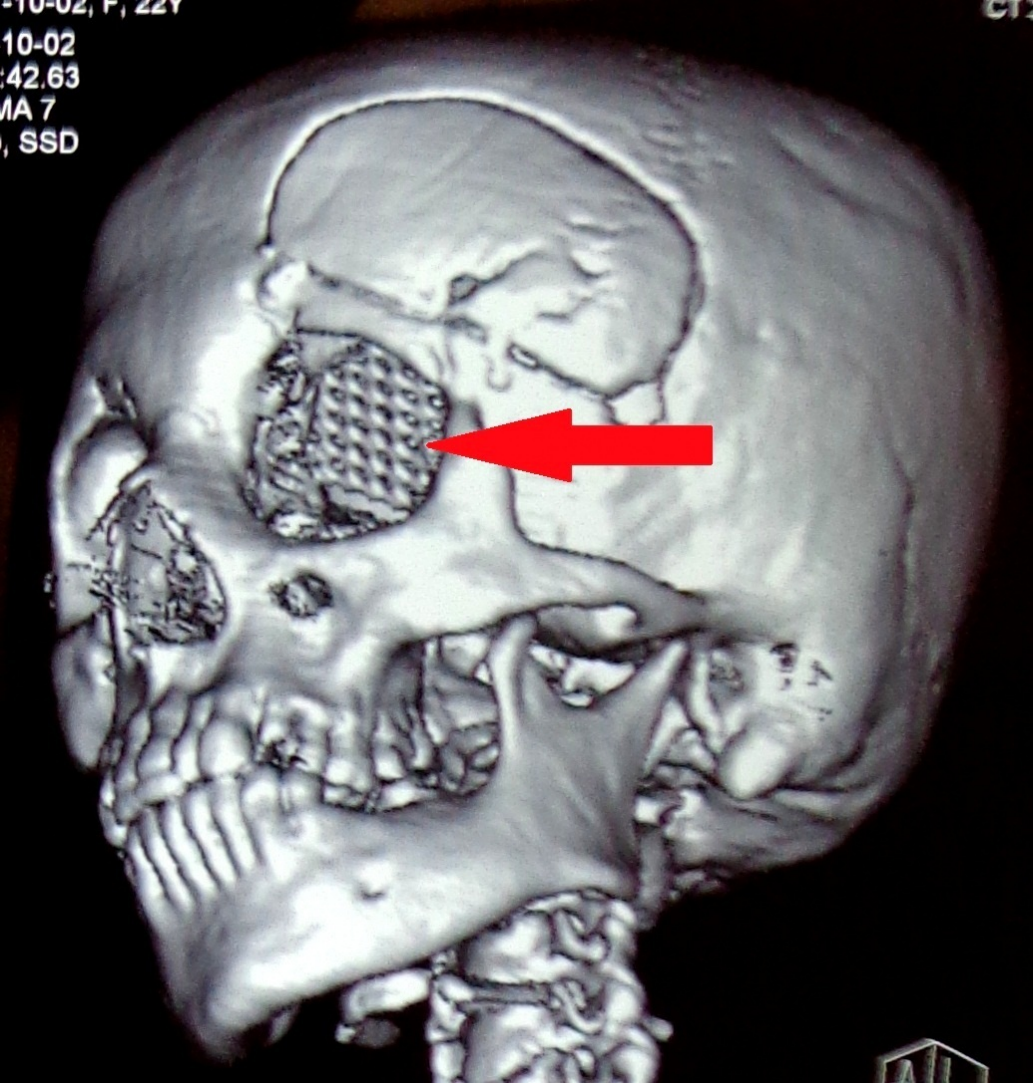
Post-operative CT, 3D reconstruction showing metallic prosthesis seen in roof of the left orbit. 3D: Three-dimensional; CT: Computed tomography.
